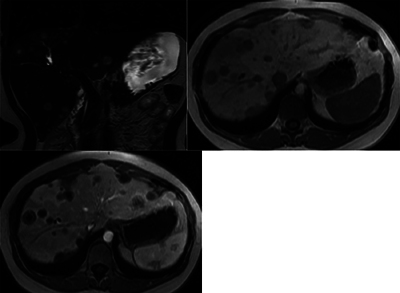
MRI showing multiple, coalescent liver lesions with target appearance, peripheral enhancement and central necrosis (upper left: T2WI; upper right: T1WI; lower left: T1WI + Gd).
